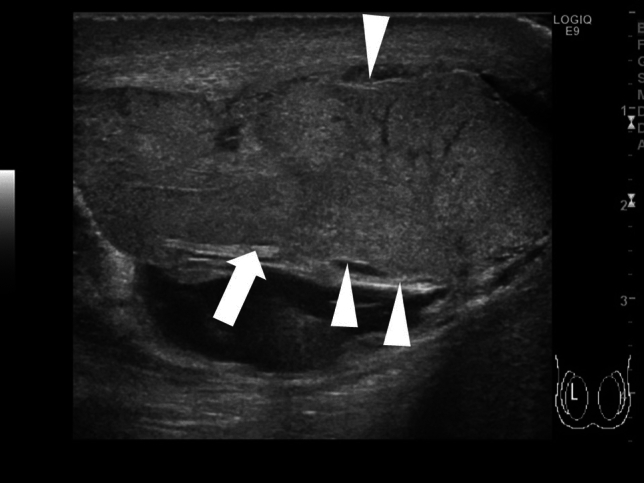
Ultrasonographic images of a 14-year-old boy with right testicular trauma and testicular rupture. The tunica albuginea is damaged in the upper pole
